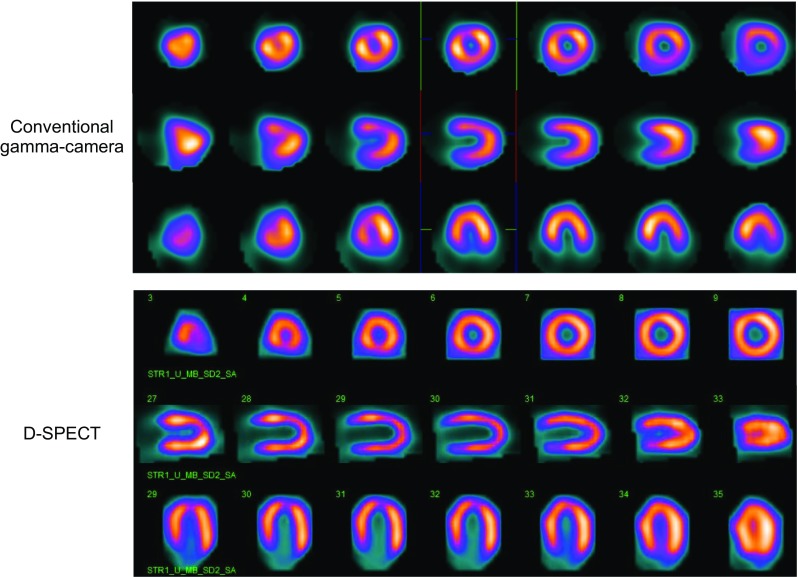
Comparison between stress MPS images of a 48-year-old woman with hypertension, dyslipidemia and familiarity for CAD acquired with two different gamma cameras. The images acquired with a conventional dual-head GP gamma camera reveal a defect in anterior wall due to attenuation by left breast (*top*), while the stress images with a dedicated CZT gamma camera (D-SPECT) and upright patient position show homogenous left ventricular perfusion (*bottom*)
